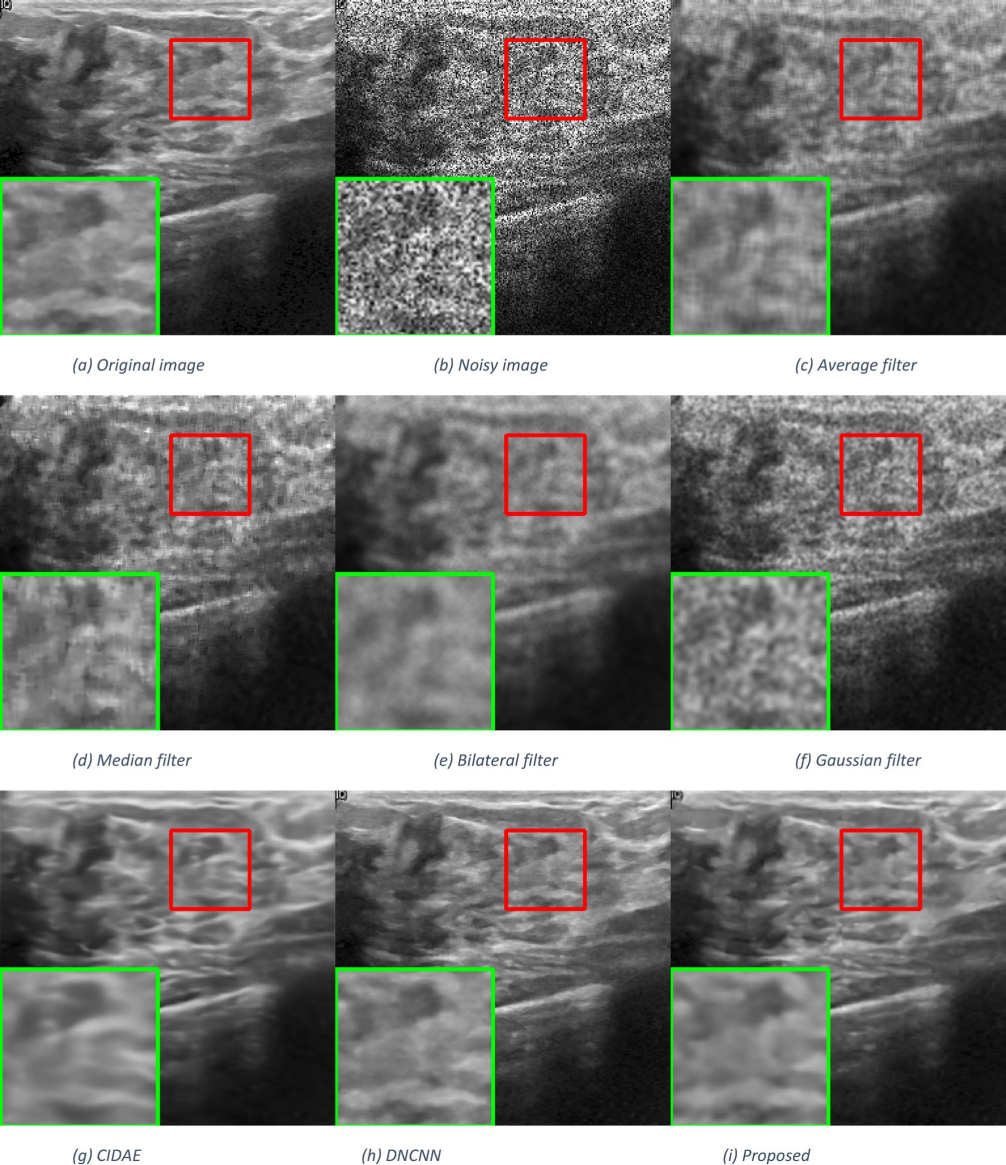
Qualitative comparisons of the proposed method with other despeckling methods on a breast ultrasound image at noise standard deviation σ = 0.25.
